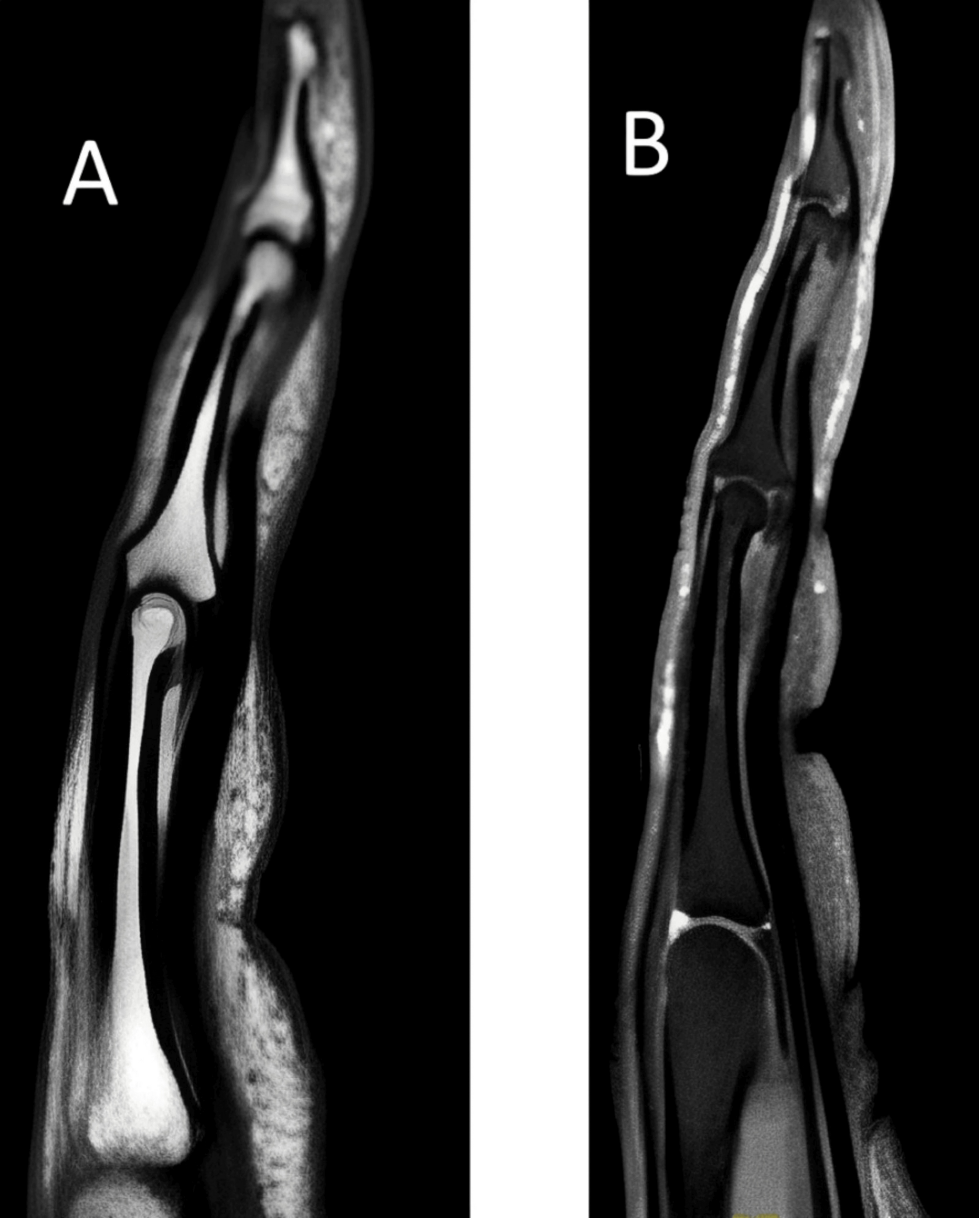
Normal MRI of the middle finger (A) T1-weighted image showing no lesion. (B) T2-weighted image showing no lesion.
